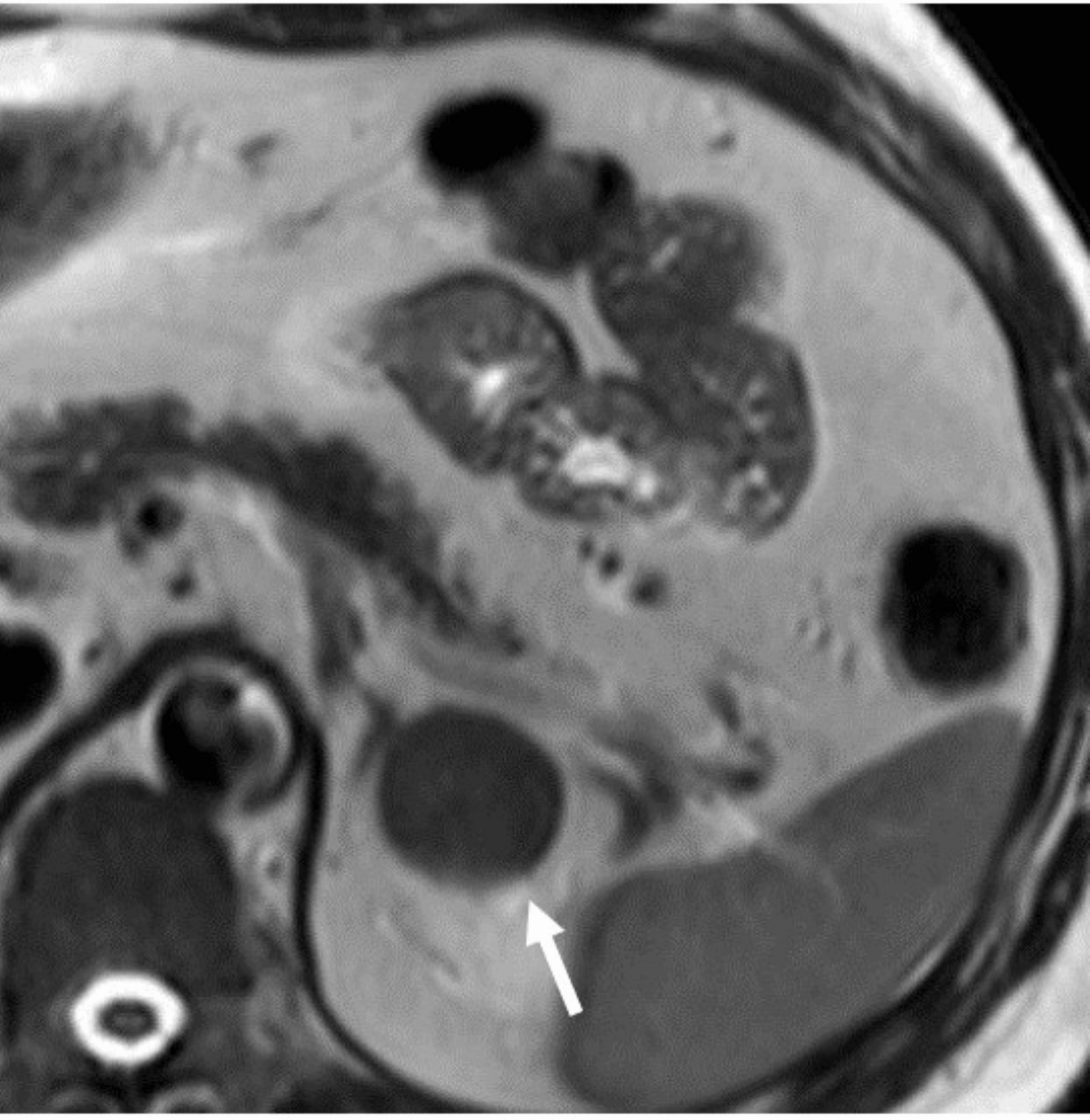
Axial long TE, T2-weighted MRI for a 33-year-old man with incidental adrenal adenoma showing homogeneous signal intensity (white arrow) TE: time echo, MRI: magnetic resonance imaging
